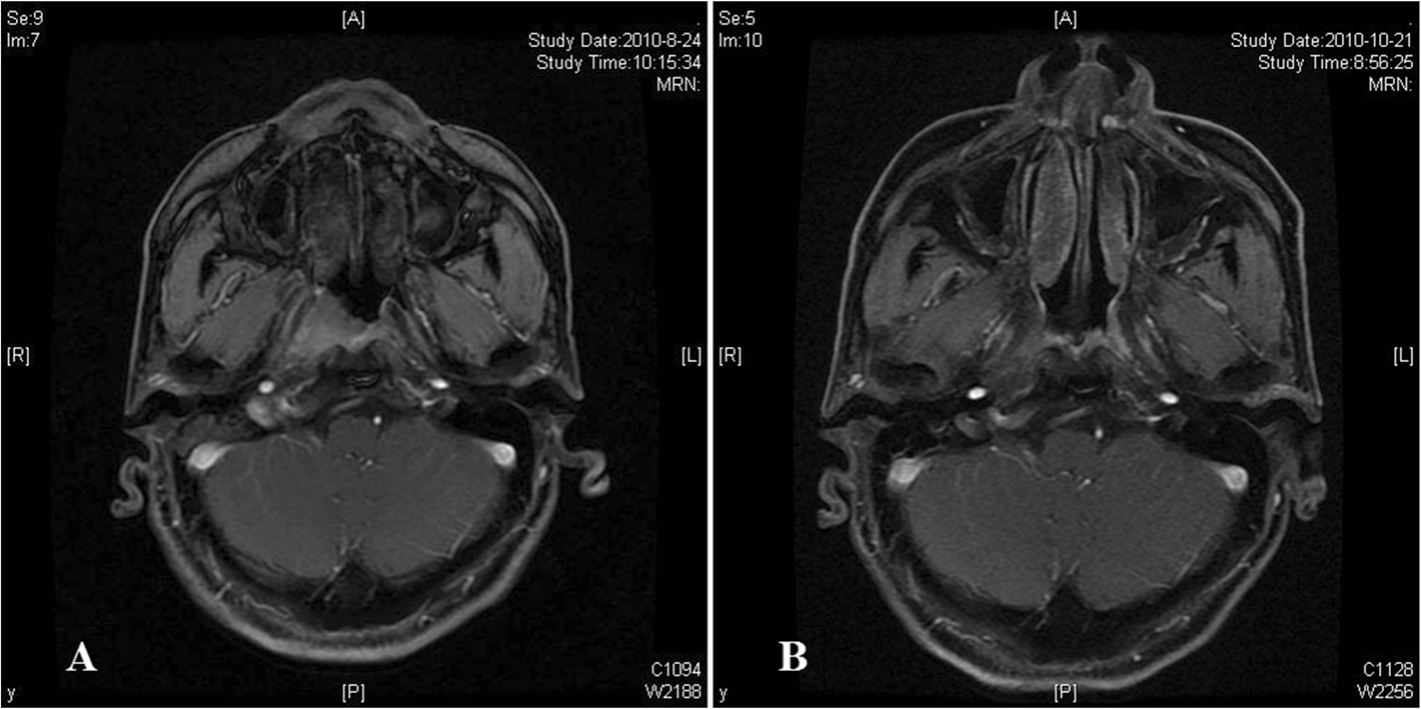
Axial contrast-enhanced MRI scan. a, MRI scan (2010.8.14) reveals a tumour in the right posterior nasopharynx that extends into the right parapharyngeal space and into the medial pterygoid muscle. b, MRI scan after radiotherapy (2010.10.21) reveals no residual tumour at the primary site
